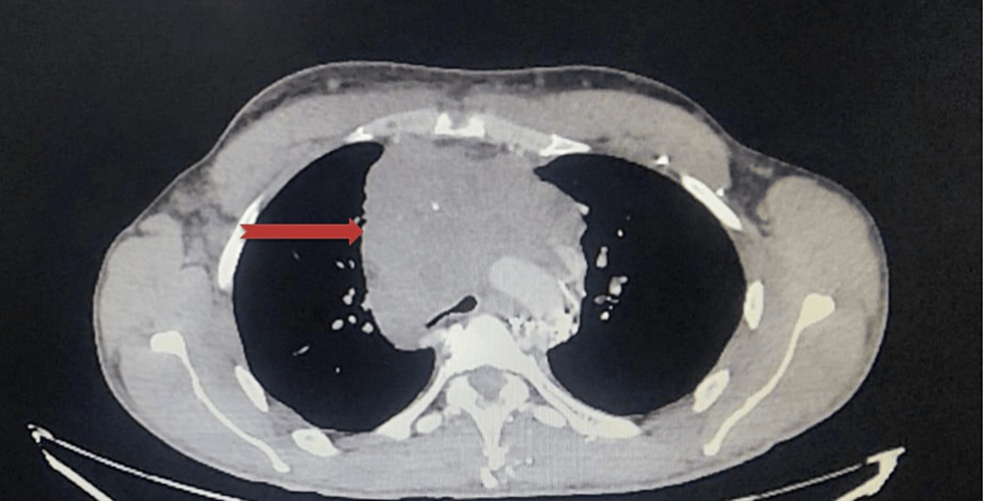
Thoracic CT scan showing large anterosuperior mediastinal tumor enveloping vital mediastinal structures (red arrow)
